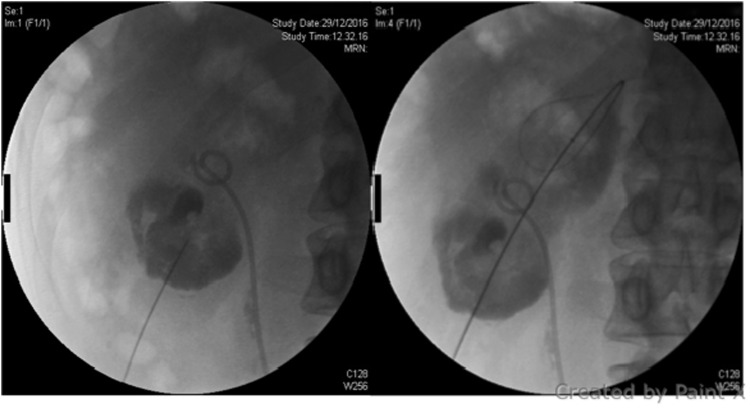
Subcapsular kidney collection puncture and mono-J drain placement under sonographic and radiologic guidance as performed by the interventional radiologist.
